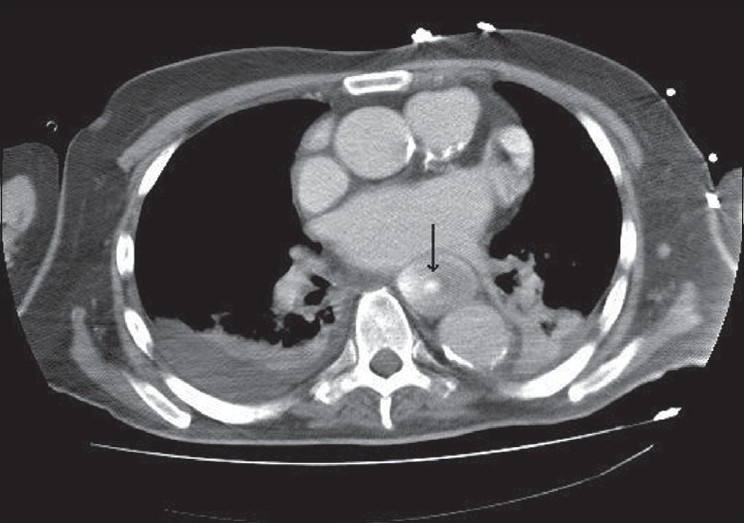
Chest CT scan showing intra-luminal space occupying lesion in the esophagus
